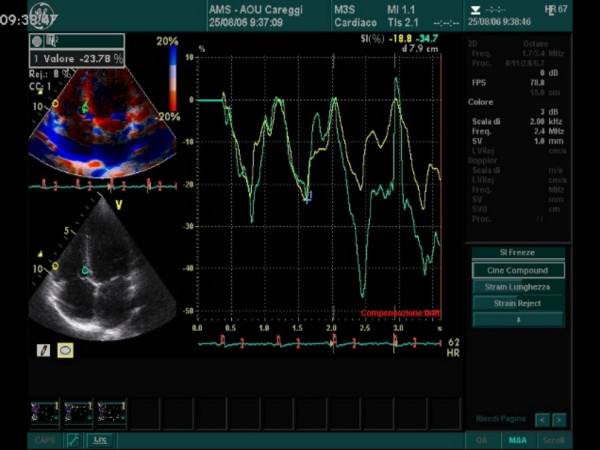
This panel shows right ventricle interventricular septum (IVS) and lateral wall (LW) systolic peak strain values with Tissue Doppler. All regions are located in basal segments.
